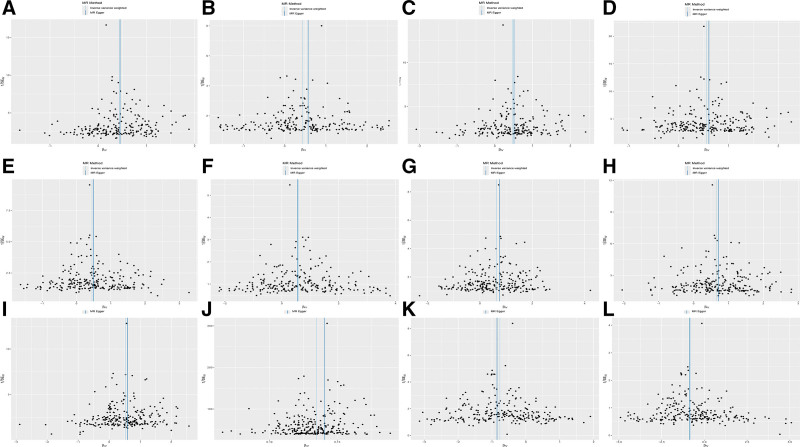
Funnel plots. Funnel plot for the effect of heel broadband ultrasound attenuation, direct entry on (A) femoral neck BMD, (B) forearm BMD, (C) lumbar spine BMD, (D) total body BMD, (E) total body BMD (0–15), (F) total body BMD (15–30), (G) total body BMD (30–45), (H) total body BMD (45–60), (I) total body BMD (over 60), (J) heel BMD, (K) osteoporosis, (L) osteoporosis fracture. BMD = bone density, MR = Mendelian randomization.
